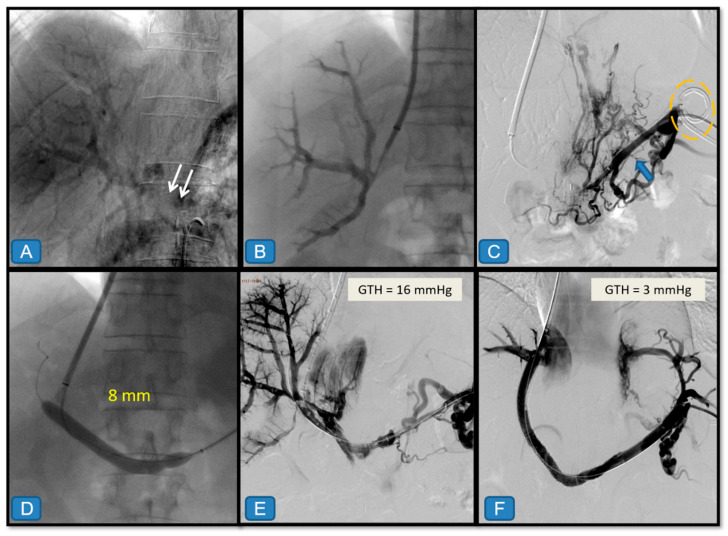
Combined transsplenic approach in a 49-year-old man with history of pancreatic surgery, prehepatic portal hypertension with stenosis of the portomesenteric anastomosis and portal cavernomatosis. (A) Indirect portography shows chronic thrombosis of the entire superior mesenteric vein extending through the main portal trunk and the splenomesenteric portal venous confluence (white arrows), with the right and left portal branches remaining patent. (B) Through standard transjugular access, direct portography confirmed chronic thrombosis of the entire portal trunk, which could not be successfully recanalized anterogradely despite attempts with various guidewires. (C) Under ultrasound guidance, a 10 F catheter was placed in the left flank for ascitic drainage (yellow oval), followed by ultrasound-guided transsplenic puncture (21 G needle) of an intrasplenic venous branch using a 6 F introducer sheath (blue arrow). Direct splenoportography confirms chronic thrombosis of the splenomesenteric portal venous confluence, with opacification of gastroesophageal varices. The transhepatic gradient (GTH) was 16 mmHg. (D,E) The portal trunk was successfully recanalized retrogradely, predilated with a conventional 8 mm balloon, achieving partial reopening of the vessel. (F) A TIPS was created connecting the right hepatic vein with the right portal branch, with an additional 9 mm uncovered stent extending the TIPS toward the patent splenic vein. The final GTH was 3 mmHg. Finally, the transsplenic tract was embolized with cyanoacrylate.
